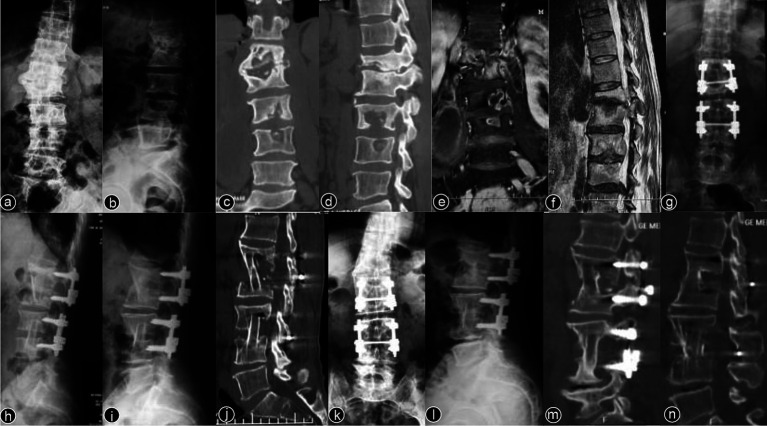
Patient, male, 38 years old. Diagnosis: spinal tuberculosis (L1–L2/L3–L4). Surgical approach: intervertebral surgery. (A,B) Preoperative anteroposterior and lateral X-rays of the lumbar spine show roughened anterior margins of the L1–L4 vertebrae and narrowing of the intervertebral spaces. (C,D) Preoperative lumbar CT reveals narrowing of the L1–L2/L3–L4 intervertebral spaces, multiple non-contiguous vertebral body destruction, and scoliosis. (E,F) Preoperative lumbar MRI demonstrates narrowing of the L1–L2/L3–L4 intervertebral spaces, abnormal vertebral signals, and an epidural abscess occupying the spinal canal. (G,H) Immediate postoperative anteroposterior and lateral X-rays of the lumbar spine. (I,J) Two-year postoperative lateral X-rays and CT scans show stable internal fixation and well-healed bone grafts. (K–N) Ten-year postoperative anteroposterior and lateral X-rays and CT scans confirm no loosening or breakage of the internal fixation, with solid bone graft fusion and no recurrence of the local lesion.
